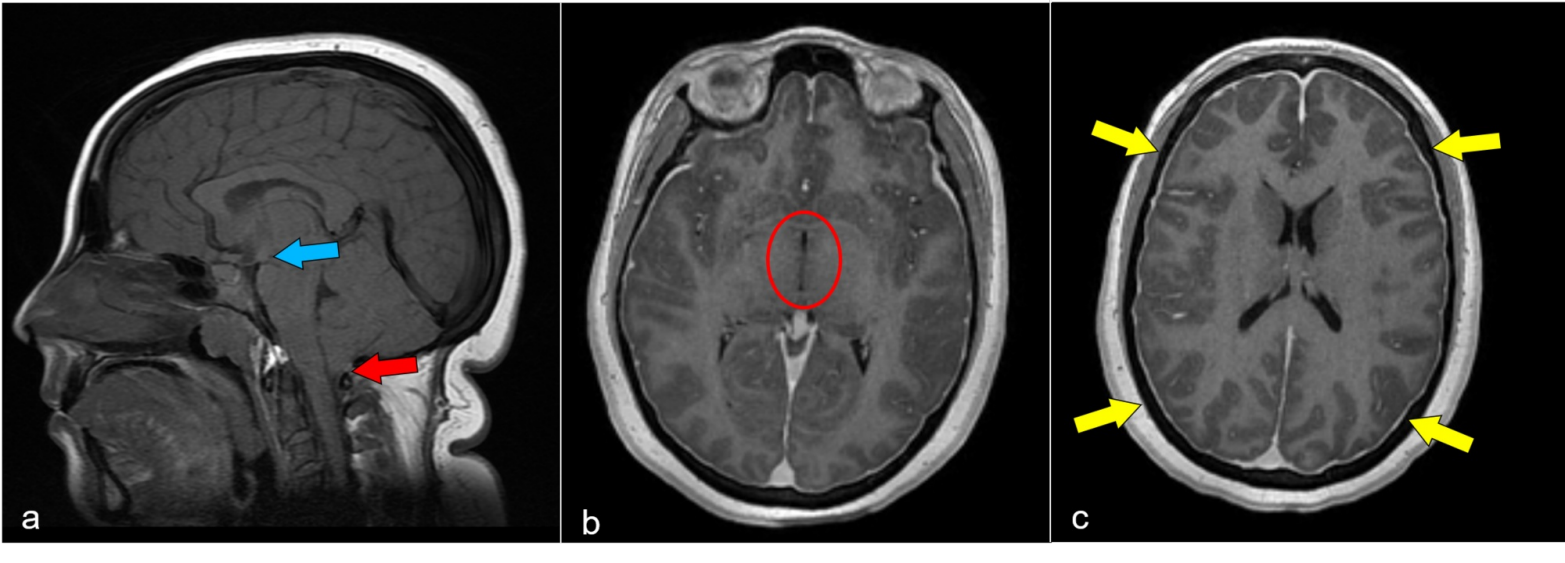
Intracranial hypotension Initial pre-operative MRI. Multiple characteristic features of intracranial hypotension are present in this patient. The sagittal non-contrast T1-weighted image (a) demonstrates mild tonsillar decent (red arrow) and crowding at the foramen magnum as well as a decreased mamillopontine distance (blue arrow). Post-contrast axial T1-weighted images (b,c) demonstrate a slit-like third ventricle (circle) and pachymeningeal enhancement (yellow arrows). MRI: magnetic resonance imaging
